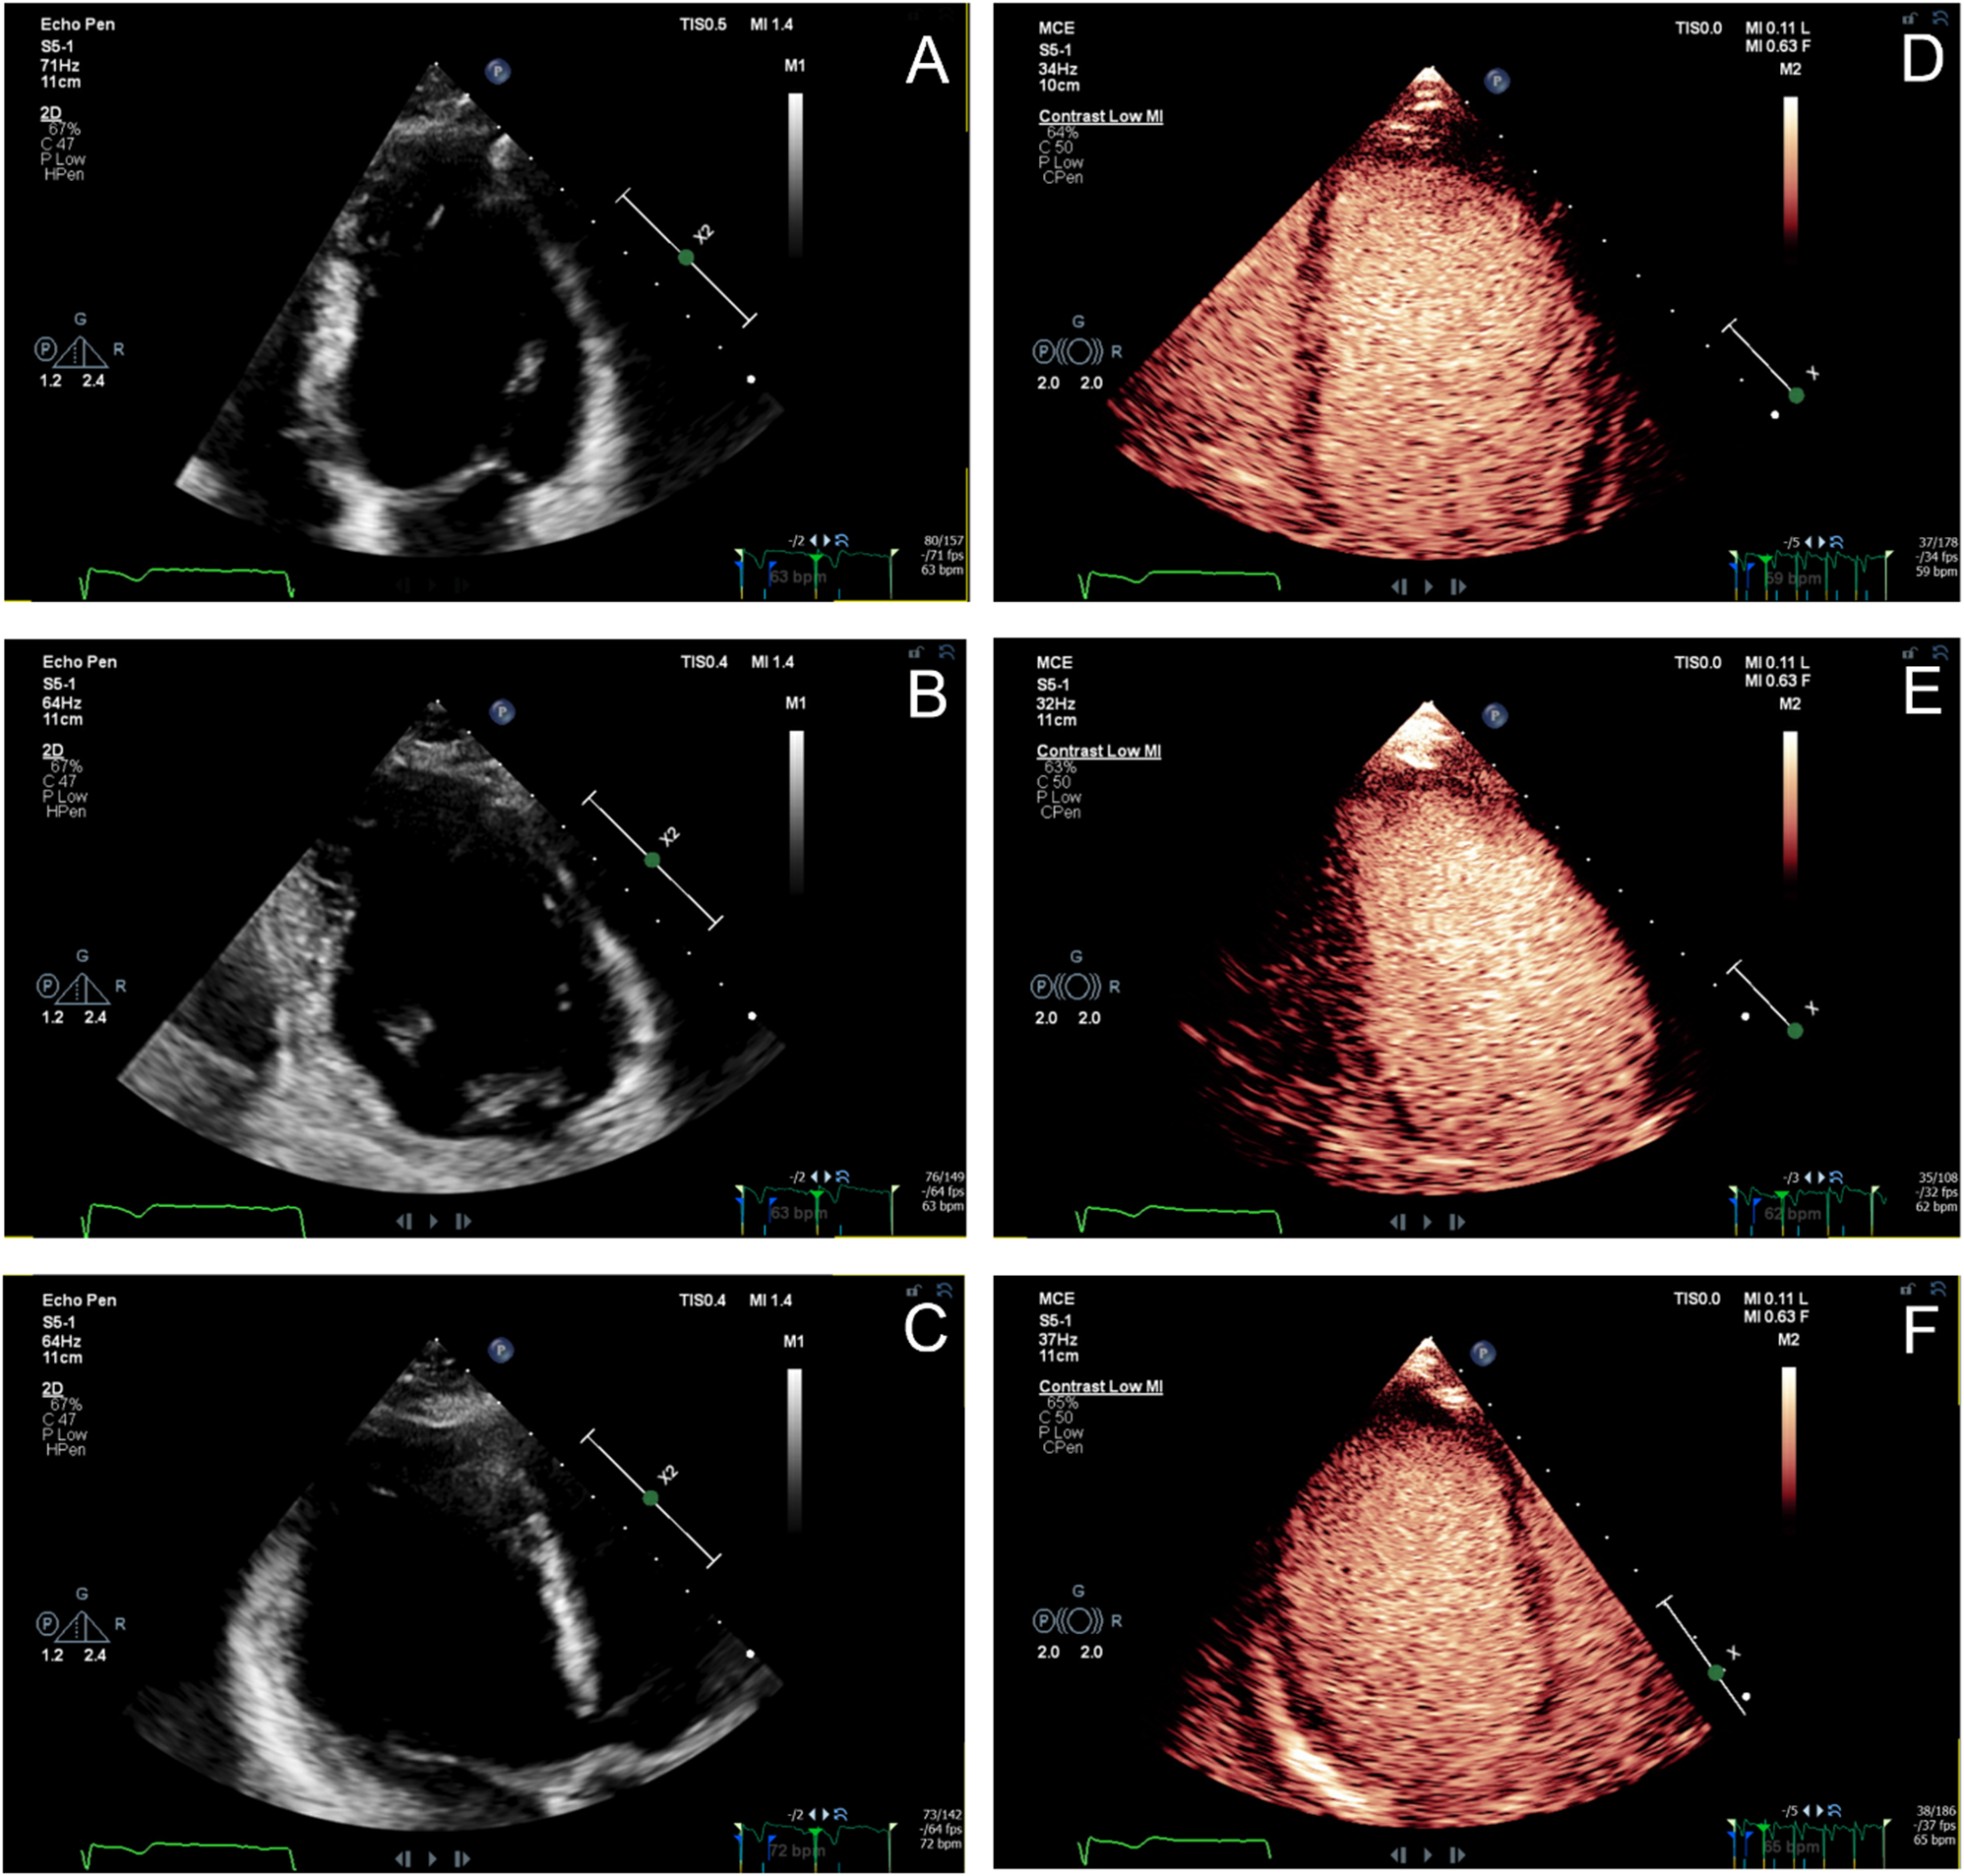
Unenhanced apical 4 chamber (A), 2 chamber (B) and 3 chamber (C) vs contrast enhanced apical 4 chamber (D), 2 chamber (E) and 3 chamber (F) at end-diastole in the same patient. Images used for the assessment of left ventricle ejection fraction for cardiac late effects in the setting of cardio-oncology
